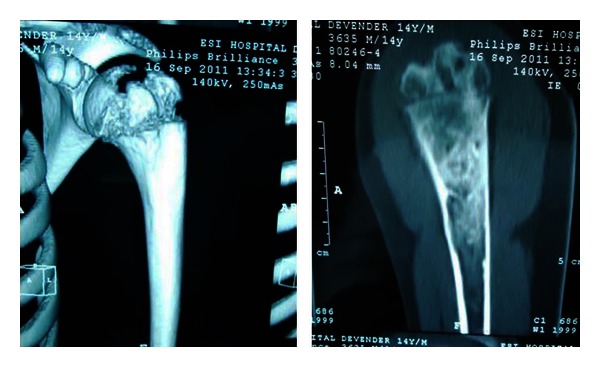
Follow-up CT scan showing healing of lesion.
